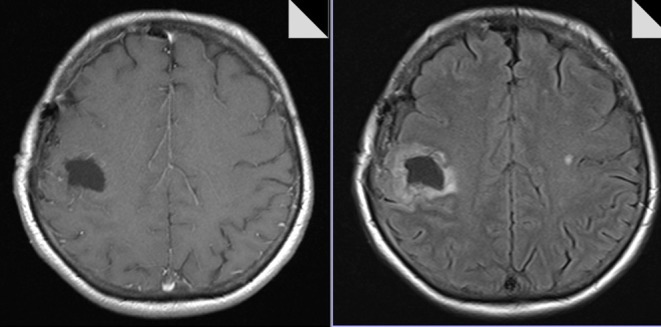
Post-operative MR image (T1-weighted and FLAIR sequences, axial planes) confirming complete removal of the tumor.
